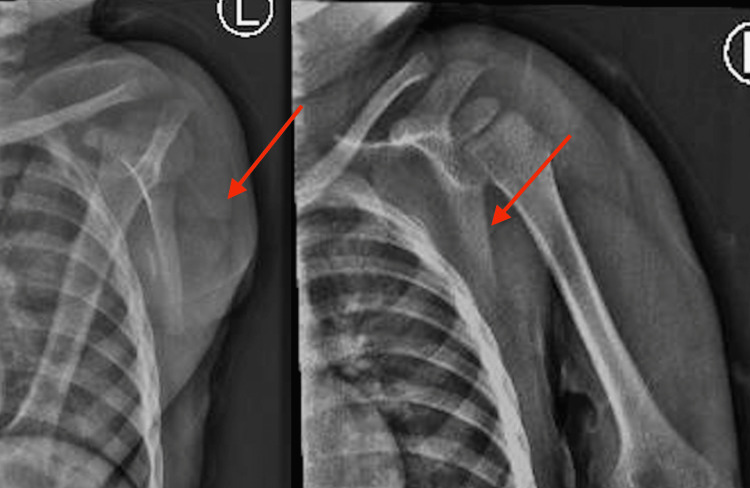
Preoperative X-ray taken for the evaluation of the patient
